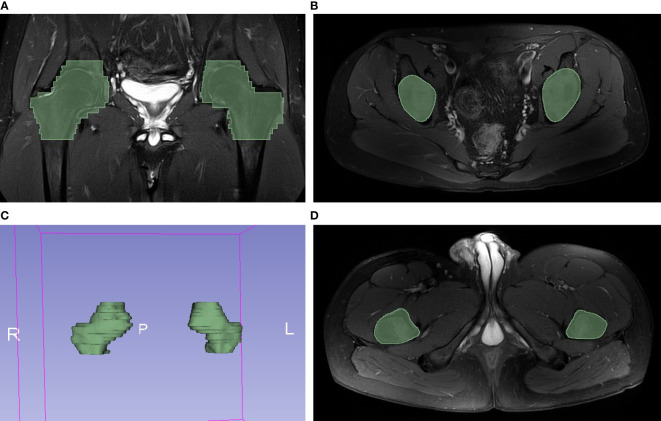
Example of hip MRI slices showed the range of handcrafted segmentation. (A) Regions of interest (ROI) of bilateral hips were labeled with green color in coronal plane. (B) The first slide containing ROI in axial plane. (C) The reconstructed 3D volume of ROI. (D) The last slide containing ROI in axial plane.
